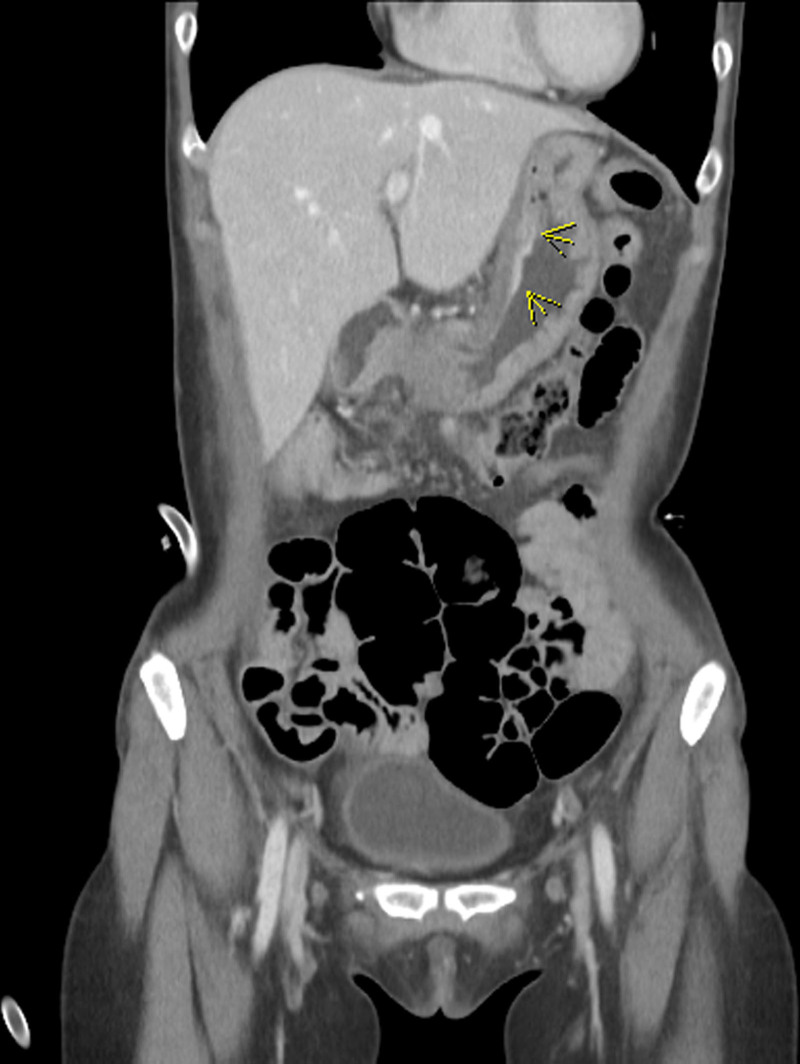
Abdominal-pelvic computed tomography (APCT) showing edematous wall thickening and suspicious mucosal hyperenhancement at the lesser curvature side of the gastric body (yellow arrow).
